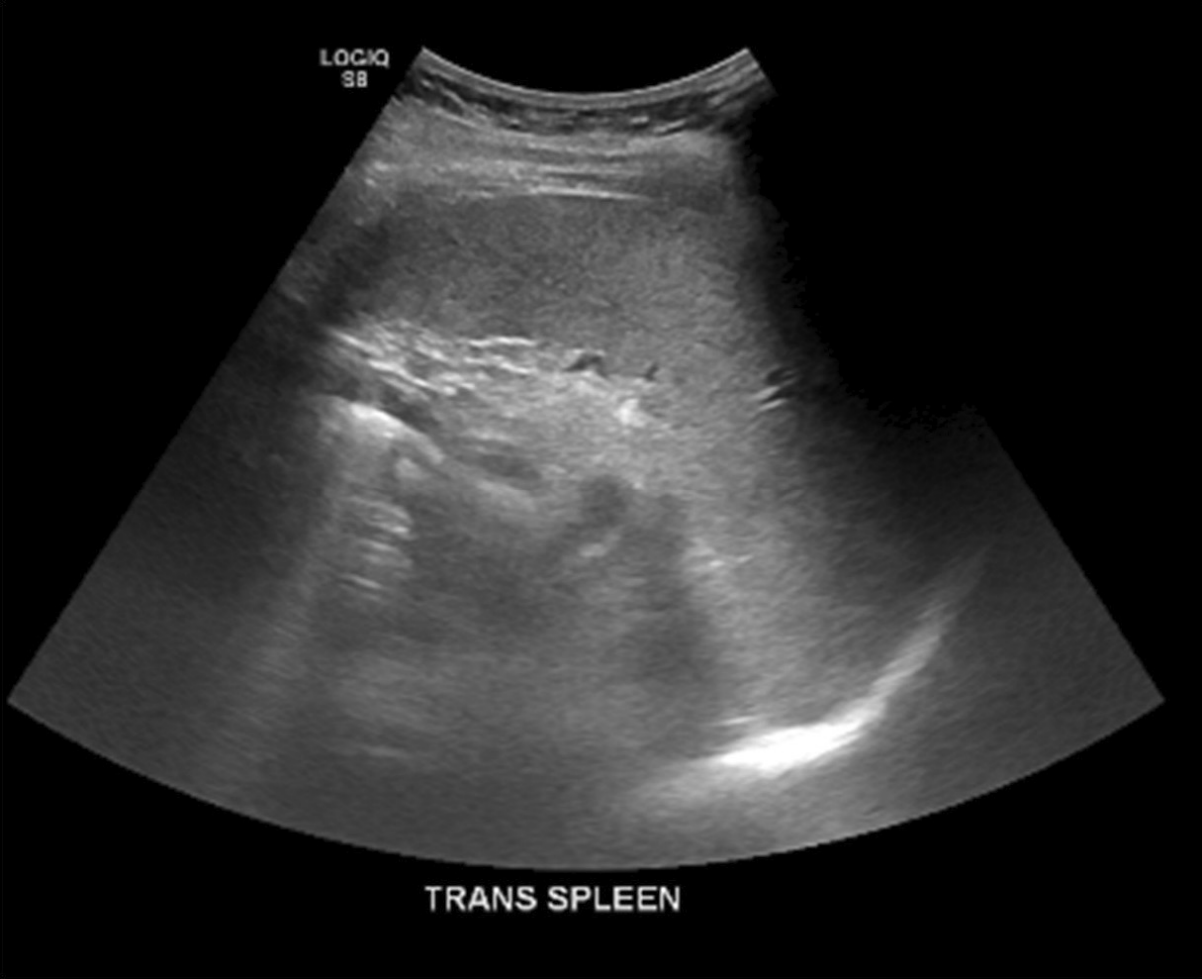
Ultrasound of the abdomen revealing a mildly enlarged spleen. This ultrasound image shows the spleen's dimensions, indicating mild splenomegaly, which is a common finding in patients with Felty Syndrome.
